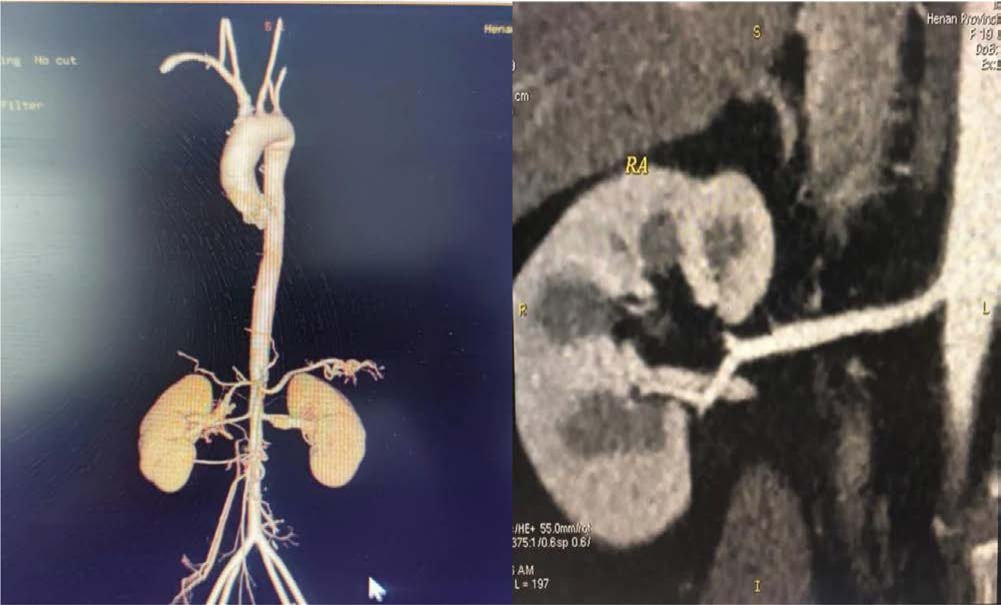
CTA was reexamined 1 yr later, no obvious stenosis. CTA = computer tomography angiography.
